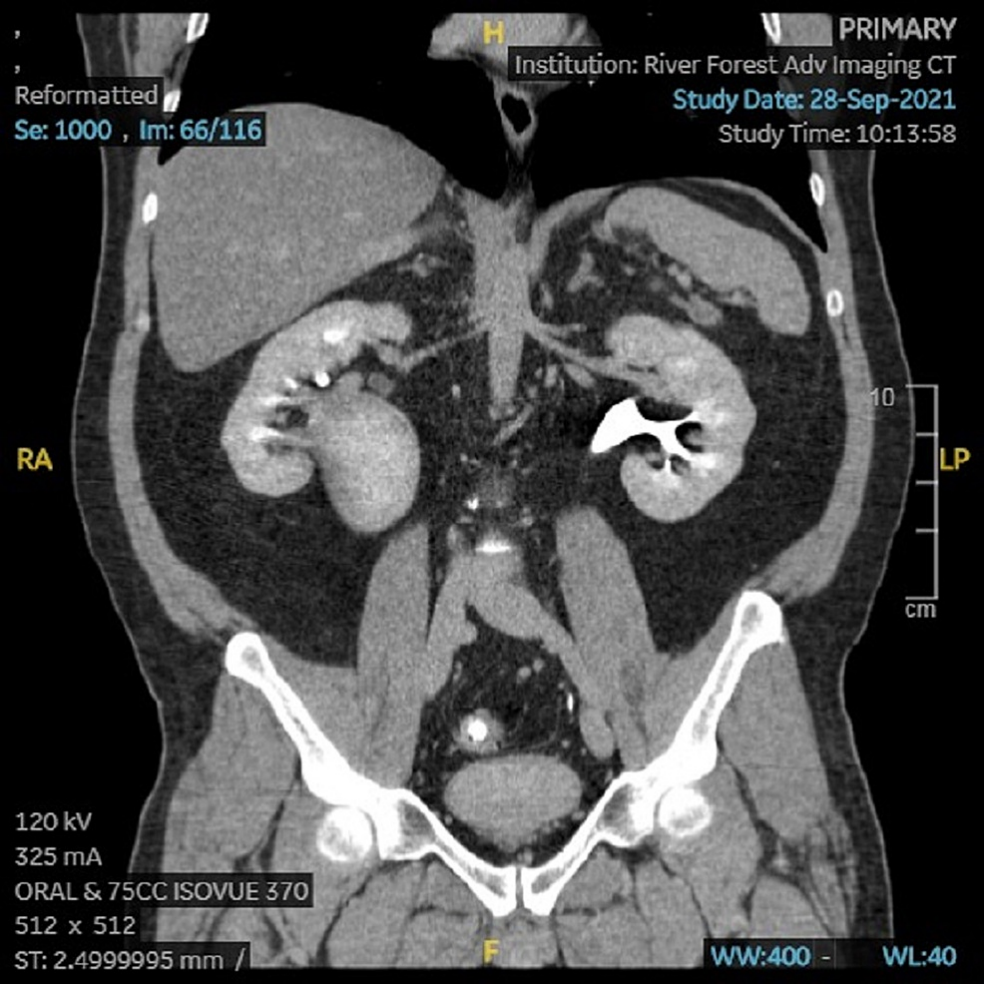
CT (coronal) image showing a very large right renal pelvis.
